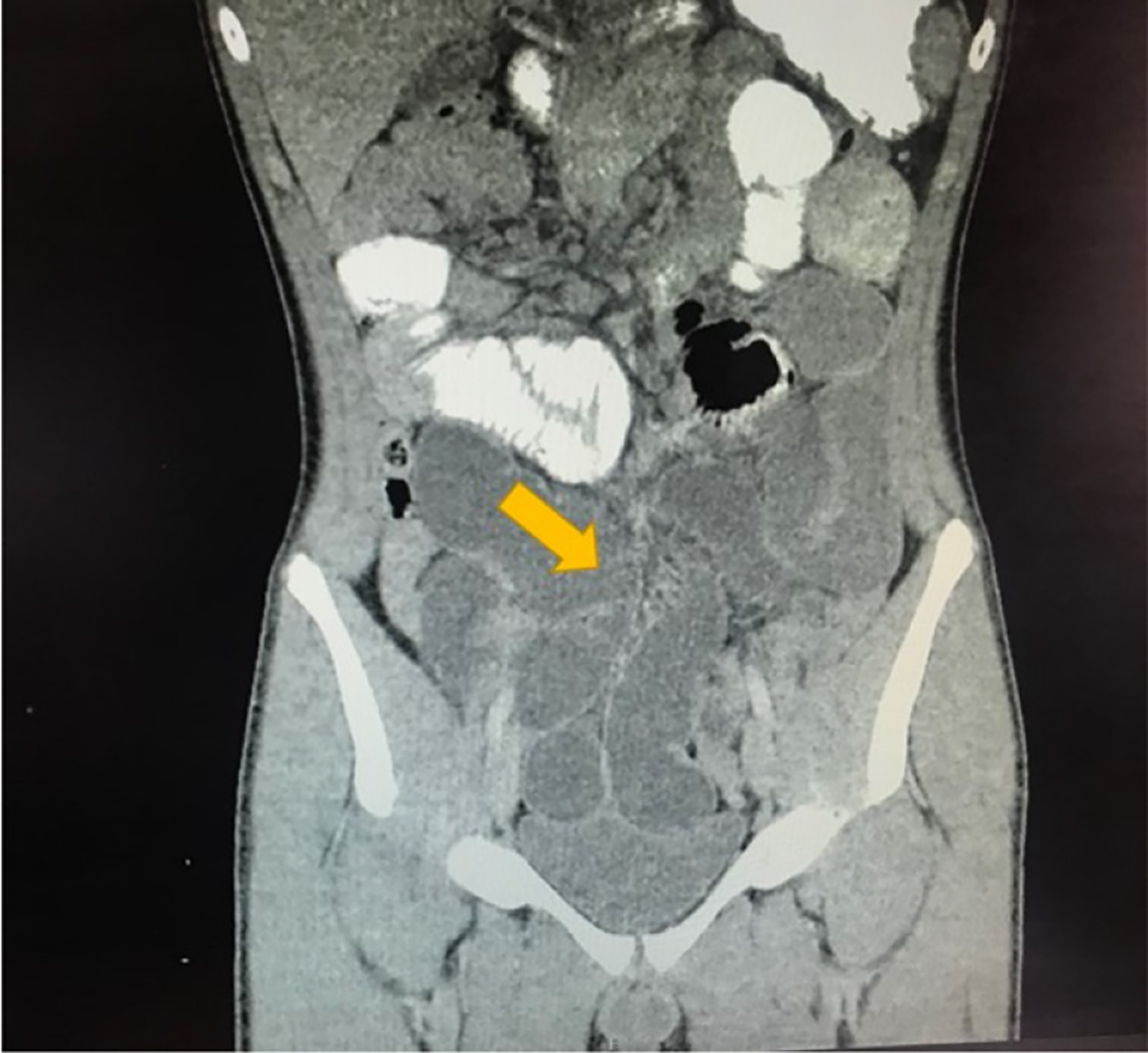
CT scan of the abdomen and pelvis with contrast showing multiple dilated loops of small bowel throughout the abdomen (arrow).
